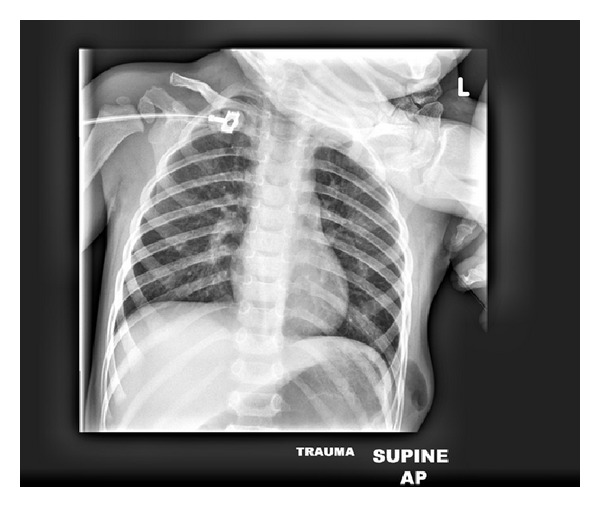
Chest radiograph reveals a tubular soft tissue lucency.
